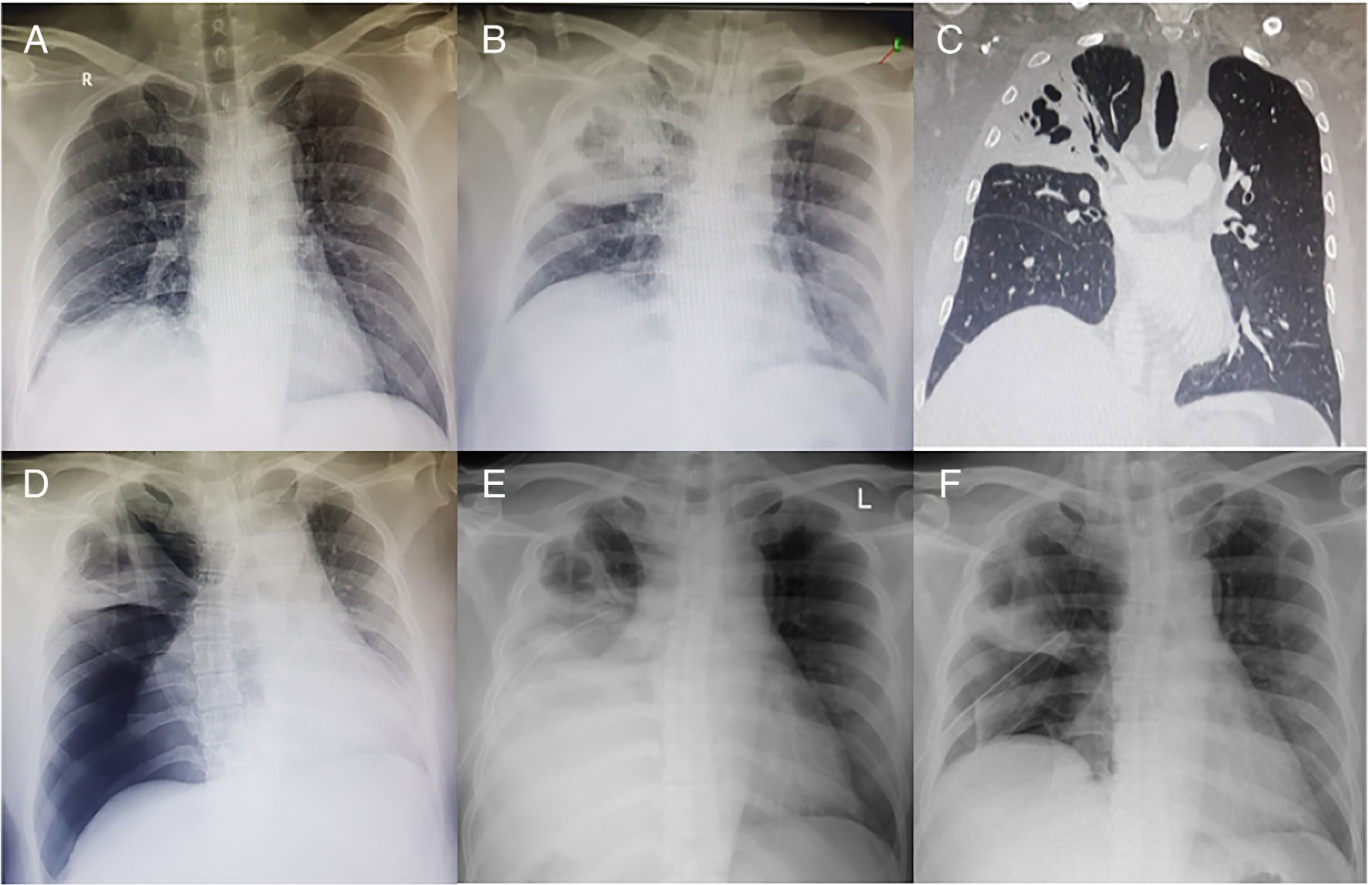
(A) Chest X‐ray that was obtained a week before the patient came to our hospital. (B) At our hospital, pre‐surgical chest X‐ray showing round shape consolidation with air space inside the right upper lung. (C) Coronal section of non‐contrast computed tomography (CT) scan showing consolidation with air bronchogram and multiple coalescence cavitary opacities at right upper lung. (D) Prior to the chest tube insertion (three days after starting tigecycline and moxifloxacin), chest X‐ray showing large pneumothorax and collapse of the right lung with contralateral mediastinal shift. (E) A day after chest tube insertion, chest X‐ray demonstrated change in air–fluid level caused by hydropneumothorax. (F) Two weeks after the chest tube insertion, chest X‐ray showing that the inflammation resolved but the lung failed to expand and the pneumothorax persists.
